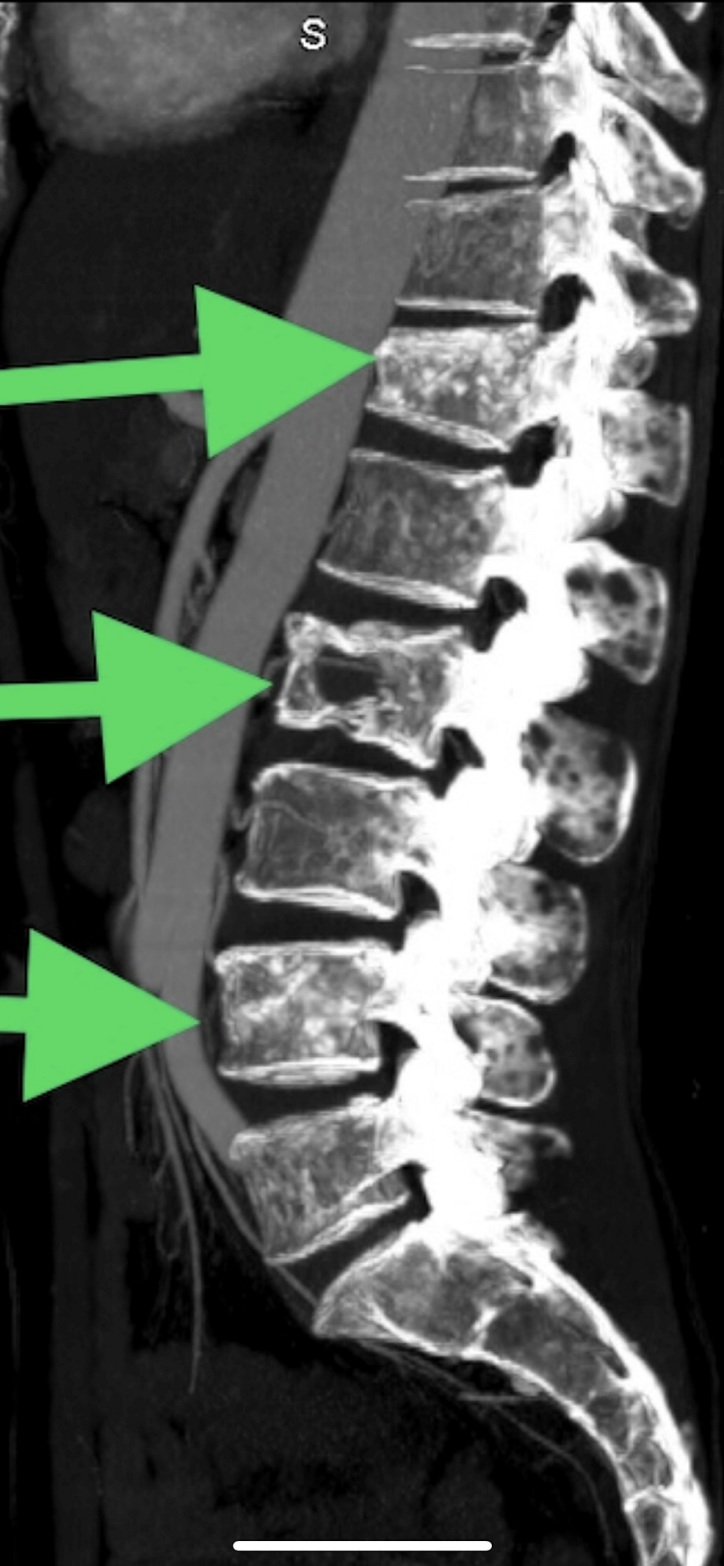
A computed tomography angiography of the abdomen (sagittal plane); the arrows indicate several lytic lesions in the spine
